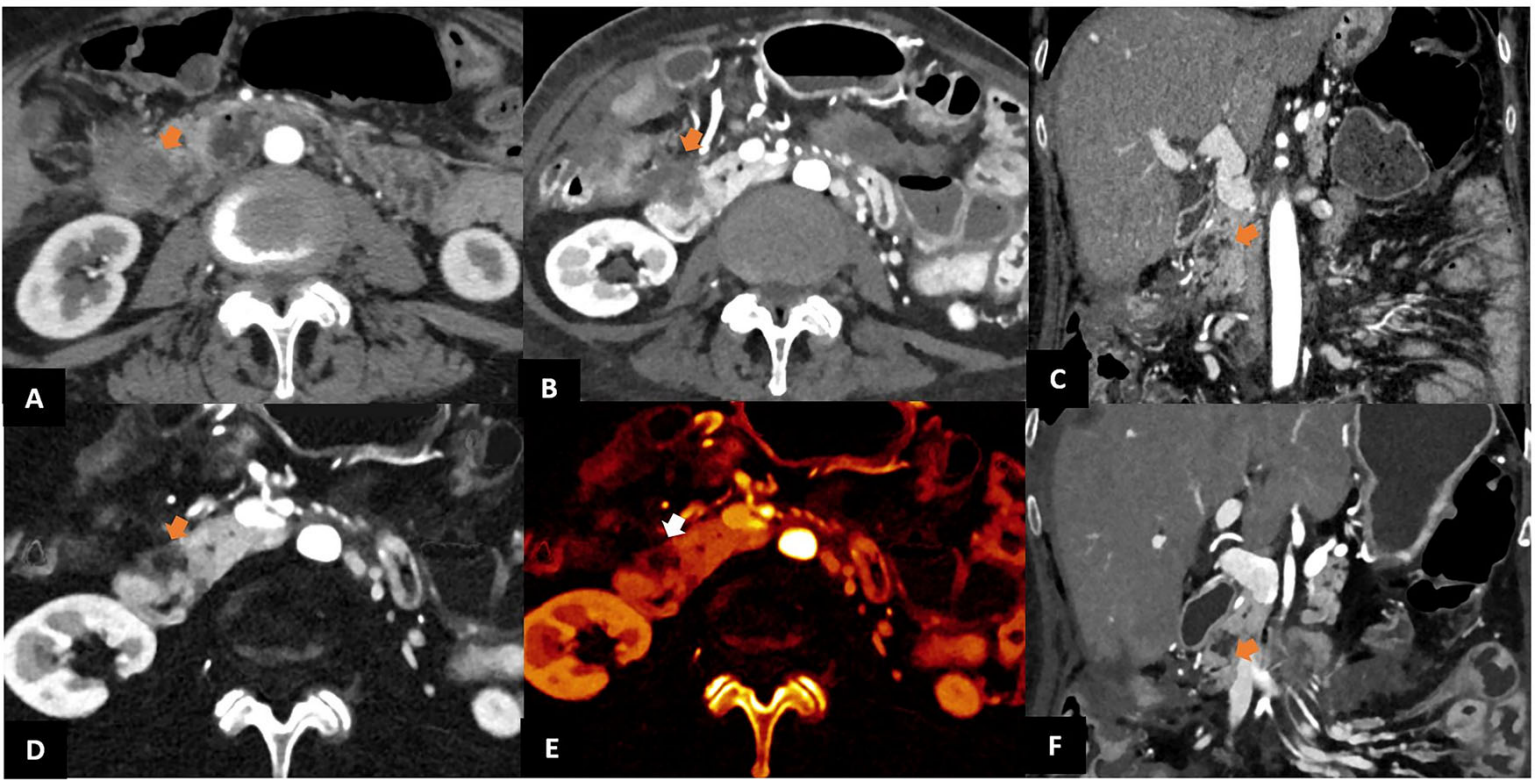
PCCT and EID-CT in pancreatic imaging. EID-CT (A) and PCCT (B) with corresponding iodine map (D) and color-coded iodine map (E) show pathological tissue measuring approximately 2.5 cm, developing from the anterior-inferior margin of the pancreatic head and infiltrating the wall of the ascending colon, the inferior duodenal flexure and the gastroduodenal artery, with better anatomical assessment on PCCT. Coronal images from EID-CT (C) and PCCT (F) demonstrate a multilocular pancreatic cystic formation. Communication with the main pancreatic duct was visible only on PCCT (arrow in F), allowing for a diagnosis of IPMN
